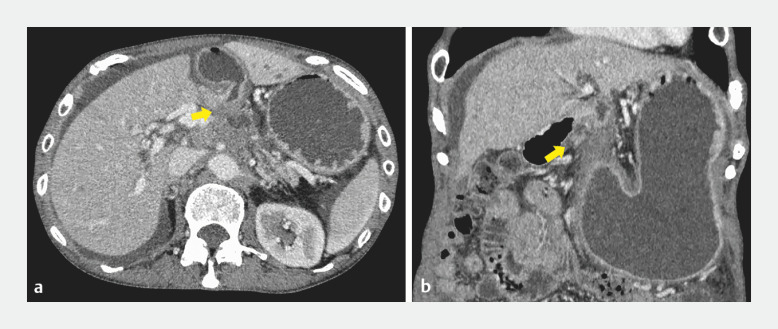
Computed tomography scan images showing gastric dilatation and stenosis (arrow) from the gastric pylorus to the duodenal bulb due to pancreatic cancer on:
